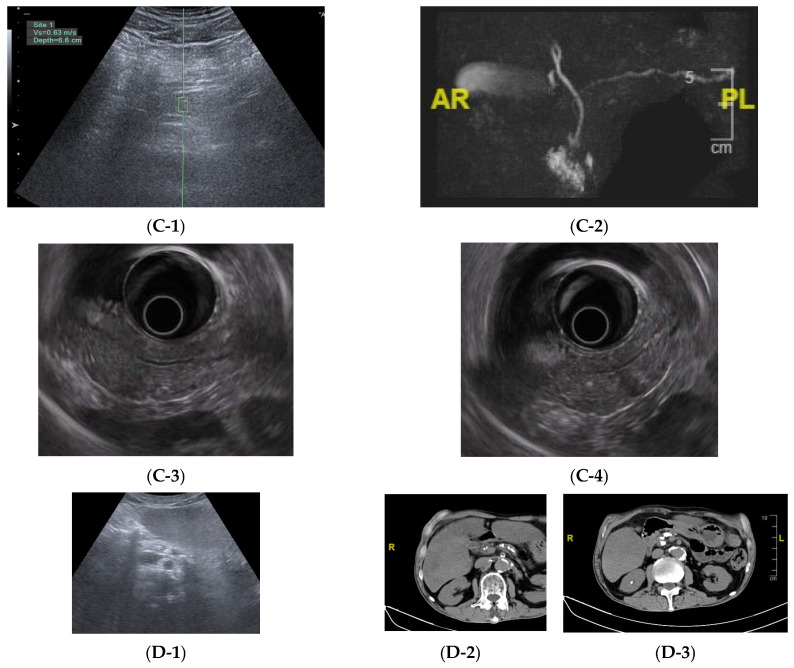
Image evidence of pancreatic fibrosis (*n* = 3) and chronic calcific pancreatitis (*n* = 1). Case 1: a 64-year-old female, abdomen sonography showed heterogenous pancreatic echotexture (A-1) and pancreatic acoustic radiation force impulse: 2.07 m/s (A-2). Case 2: a 52-year-old female, abdomen sonography showed pancreatic acoustic radiation force impulse: 1.68 m/s (B-1). Case 3: a 59-year-old man with normal pancreatic acoustic radiation force impulse (C-1) but serum CgA 121 ng/mL. The magnetic resonance image showed dilated pancreatic duct at tail part (C-2). Endoscopic ultrasonography showed pancreas fibrosis: main pancreatic duct hyperechoic edge, hyperechoic foci without acoustic shadow, dilated side branches, faint strandings (C-3,C-4); the diagnosis of pancreatic fibrosis is based on the Japan endoscopic ultrasound criteria for early chronic pancreatitis, 2019 edition [17]. A 68-year-old man with alcoholic chronic calcific pancreatitis. Typical trans-abdominal sonography (D-1) and computed tomography (D-2,D-3).
